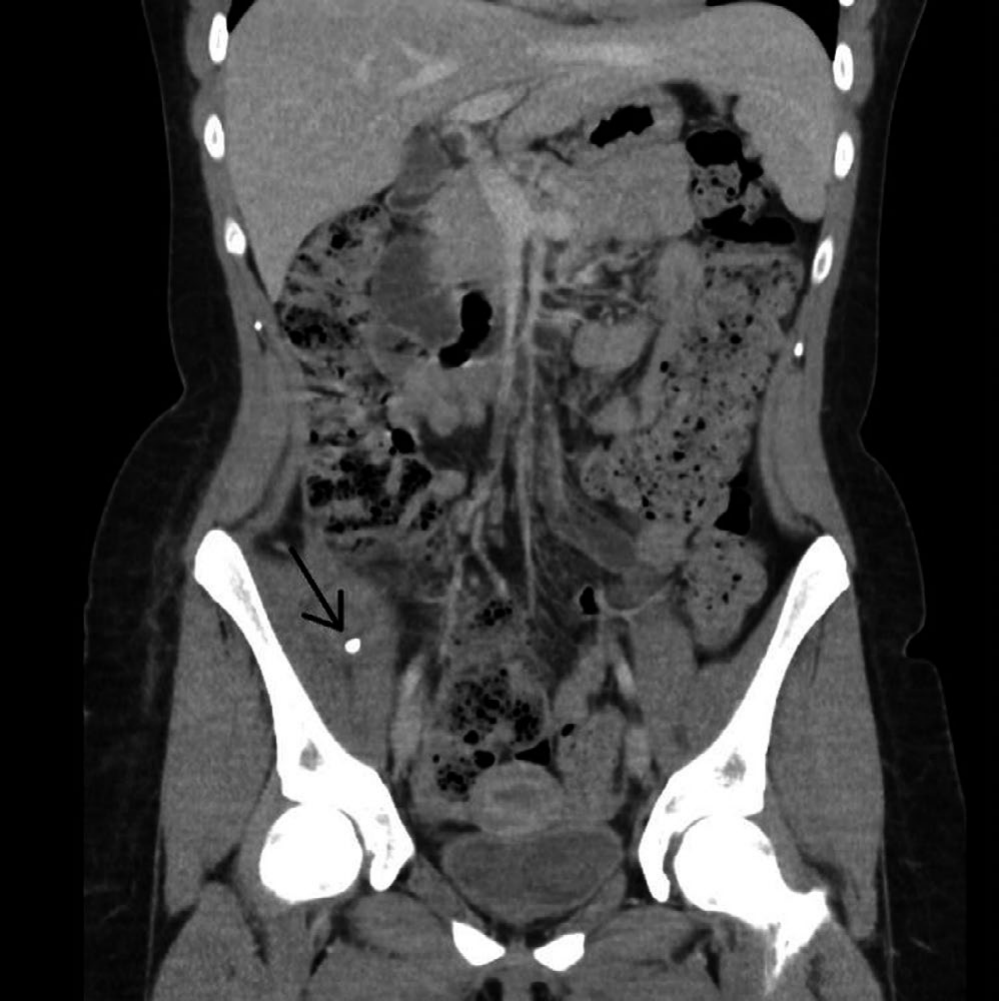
Computed tomography abdomen showing the retained fecalith
